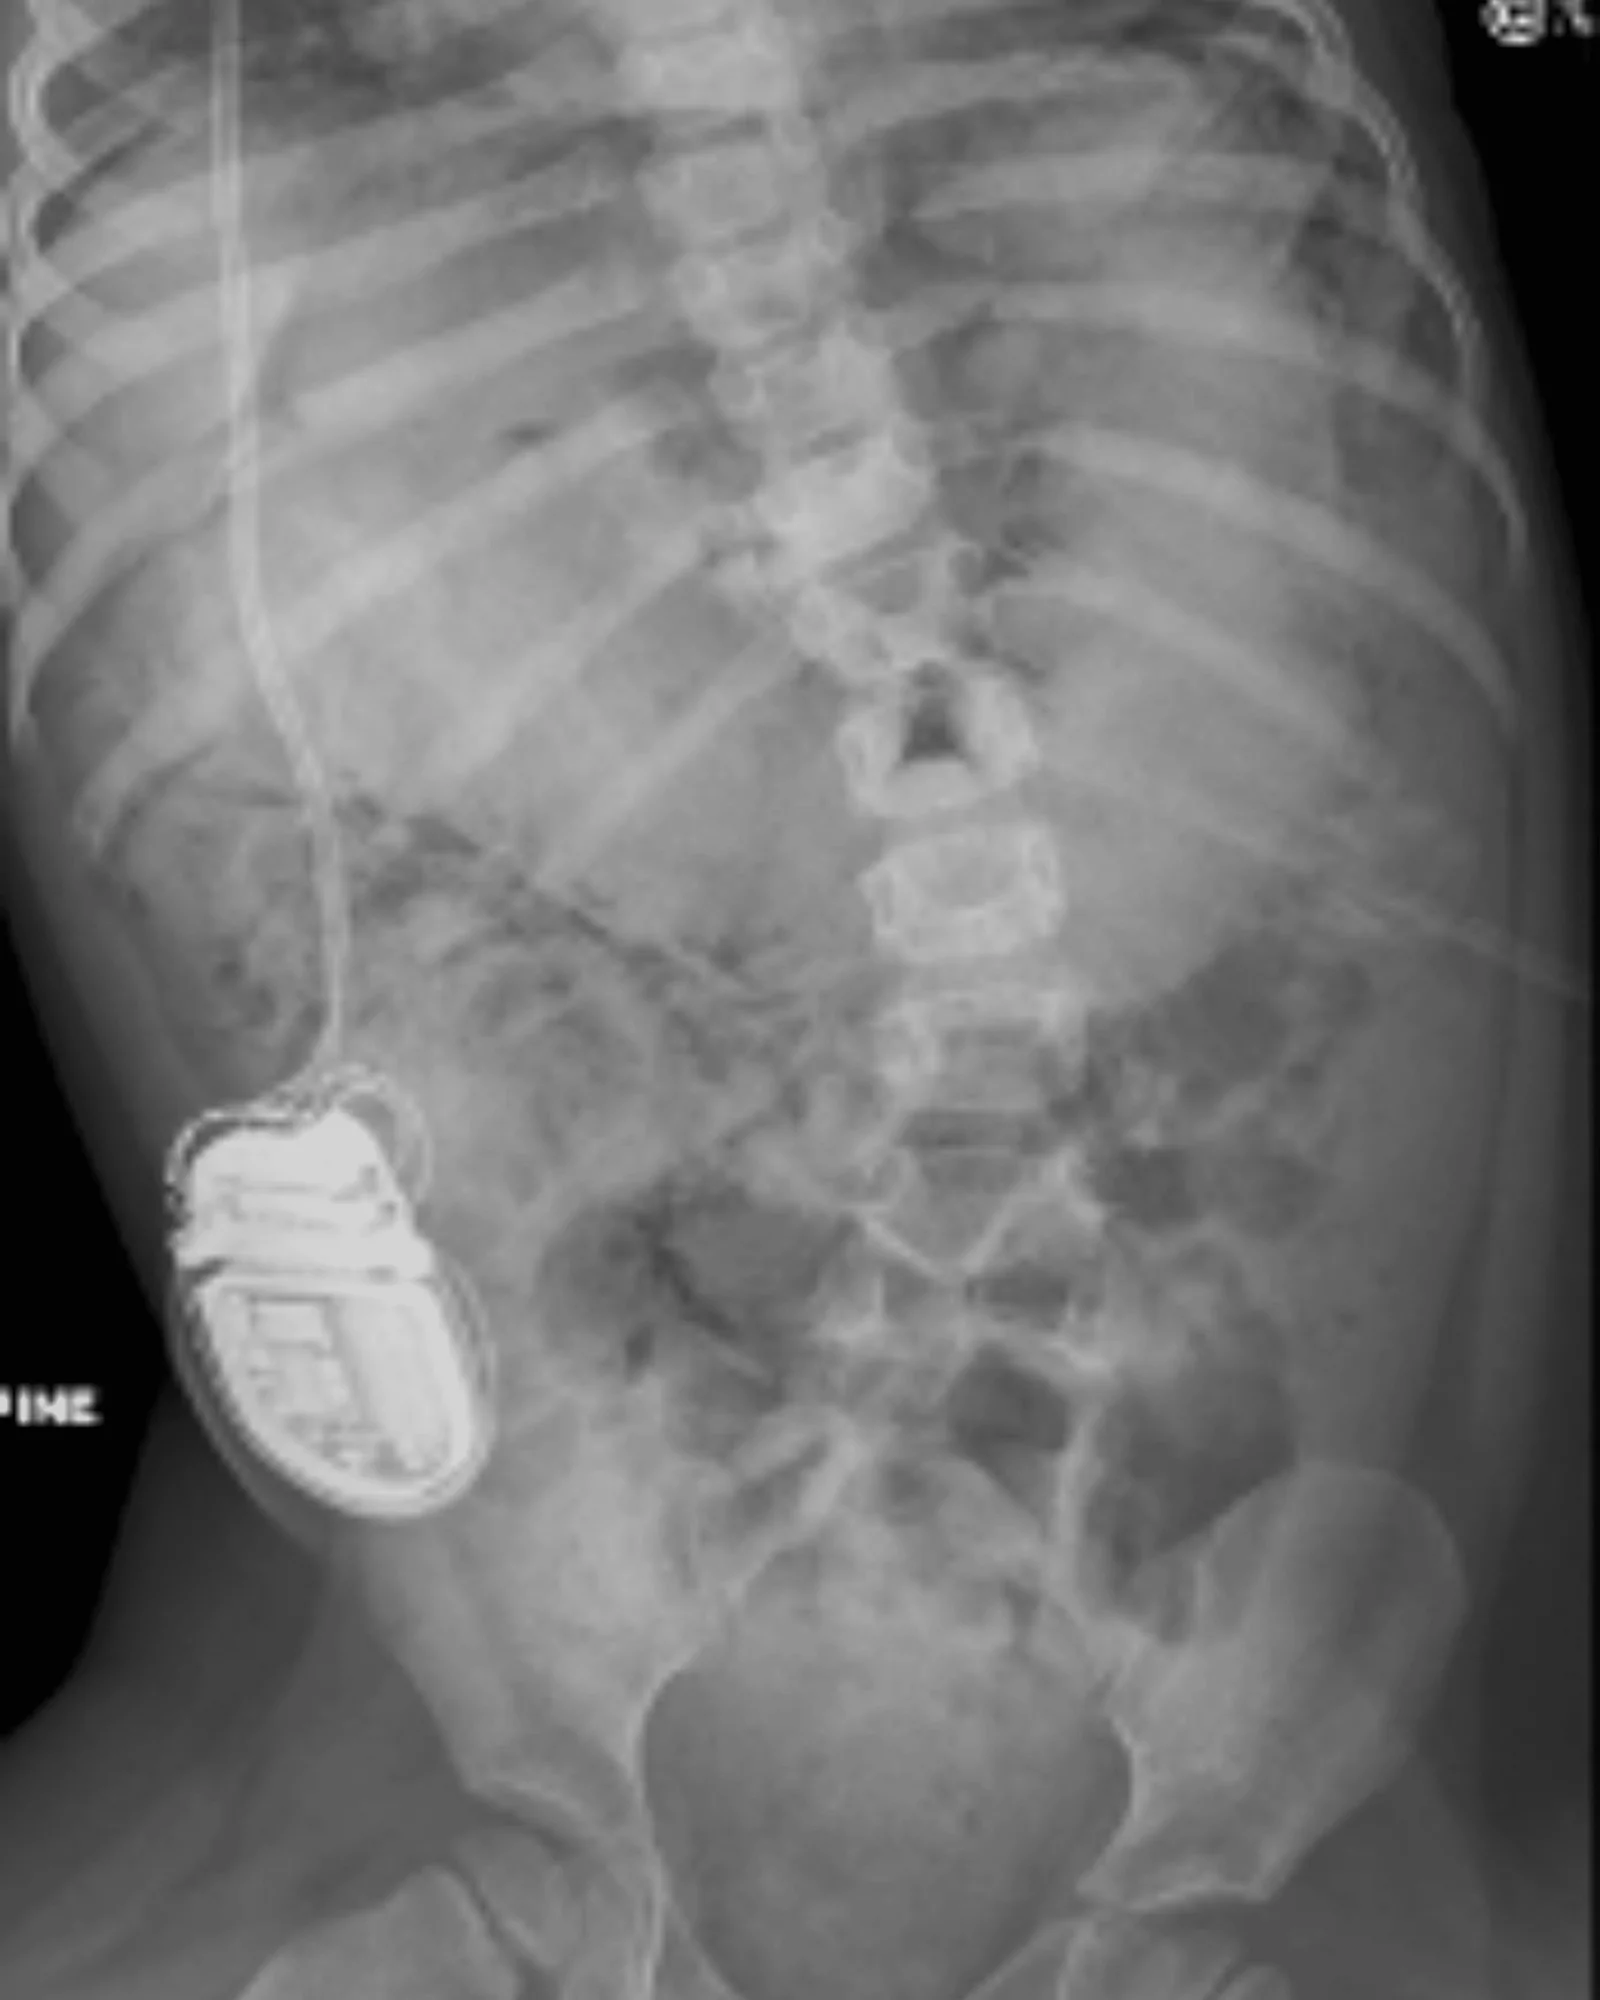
X-ray abdomen Day 3 post admission: persistent pneumoperitoneum projected over the liver, persistent impression of colonic pneumatosis, small bowel loops in the mid abdomen appear compressed, considerable amount of stool in the right transverse colon: overall improved appearance
